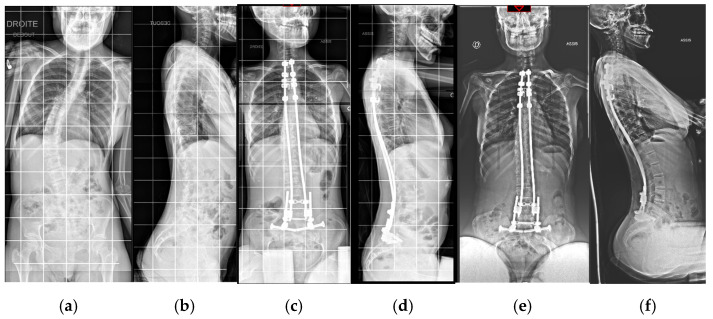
A 17-year-old girl operated on with self-expanding rods: (a,b) preoperative AP and lateral X-rays; (c,d) postoperative X-rays, (e,f) at 8 years follow-up.
